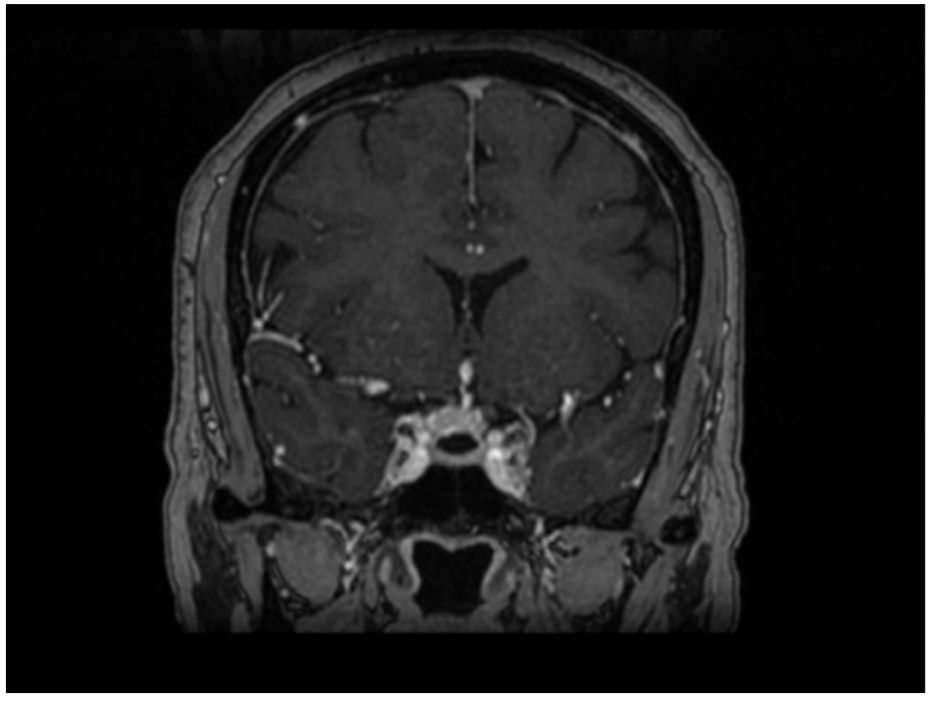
Coronal contrasted MRI showing no involvement of the cavernous sinus.
